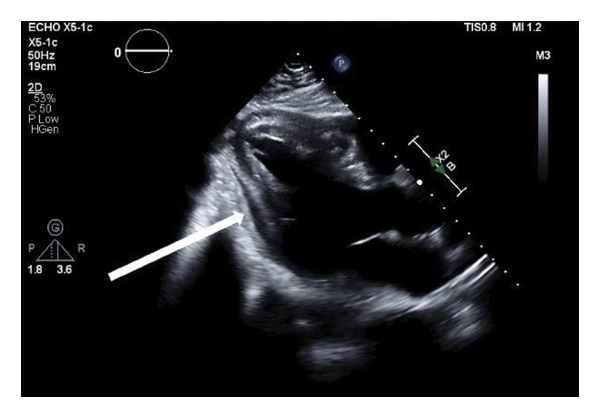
Echocardiogram: localized posterior pericardial effusion in parasternal long axis view. Caption: transthoracic echocardiogram (TTE) in the parasternal long‐axis (PSLAX) view. The white arrow identifies a localized, anechoic space posterior to the left ventricle, consistent with a trace‐to‐small pericardial effusion. This finding, identified initially via point‐of‐care ultrasound (POCUS) and confirmed by formal TTE, served as a primary diagnostic criterion for SLE‐associated pericarditis in the setting of elevated inflammatory markers.
